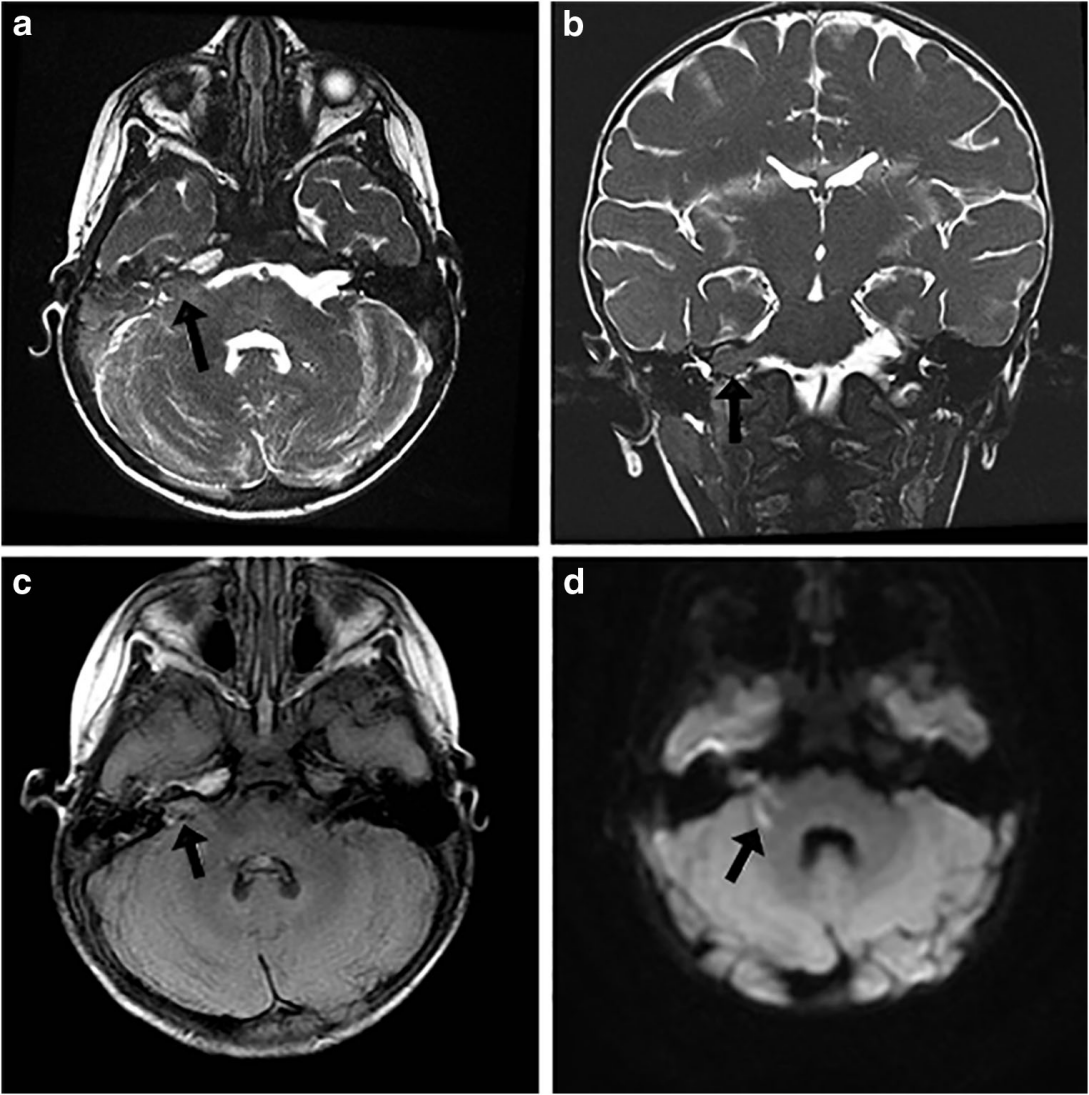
Preoperative MRI of the right cerebellopontine angle mass. a Axial and b coronal FIESTA (fast imaging employing steady-state acquisition) T2-weighted MR images showing a right cerebellopontine angle mass, with subtle T2 prolongation, inseparable with the lateral brachium pontis and extending into the internal auditory canal (arrow). c Axial T2 FLAIR (fluid attenuated inversion recovery) image demonstrates linear regions of T2 hyperintensity (arrow). d Axial diffusion-weighted image (DWI) shows linear regions of DWI hyperintensity (arrow) within the lesion (upon analysis of the apparent diffusions coefficient maps), including the component extending into the right IAC
